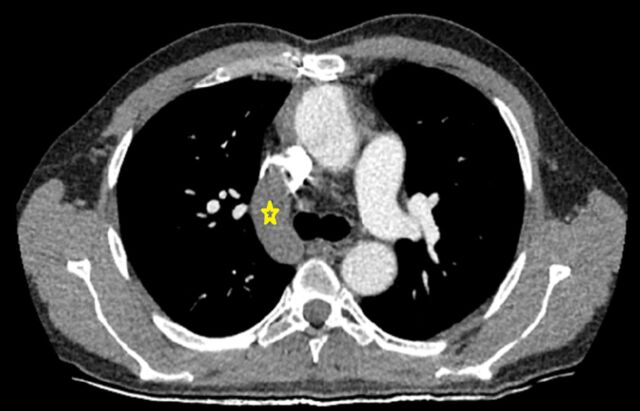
Fat-saturated contrast-enhanced computed tomography through the main pulmonary artery shows the junction of the dilated azygos vein (yellow star) and the superior vena cava.
